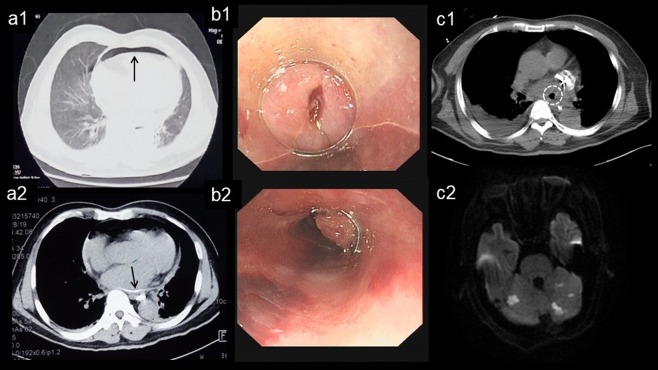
Progression from esophagopericardial fistula to atrioesophageal fistula. Unenhanced CT revealed pneumopericardium (a1, arrow) and the presence of Barium sulfate suspension prior esophagraphy within the pericardial sac (a2, arrow). Endoscopic closure of esophagus perforation with titanium clips (b1,b2). The gas (white dot circle) in left atrium documented by CT (c1) and multiple cerebral embolism documented by MRI (c2).
